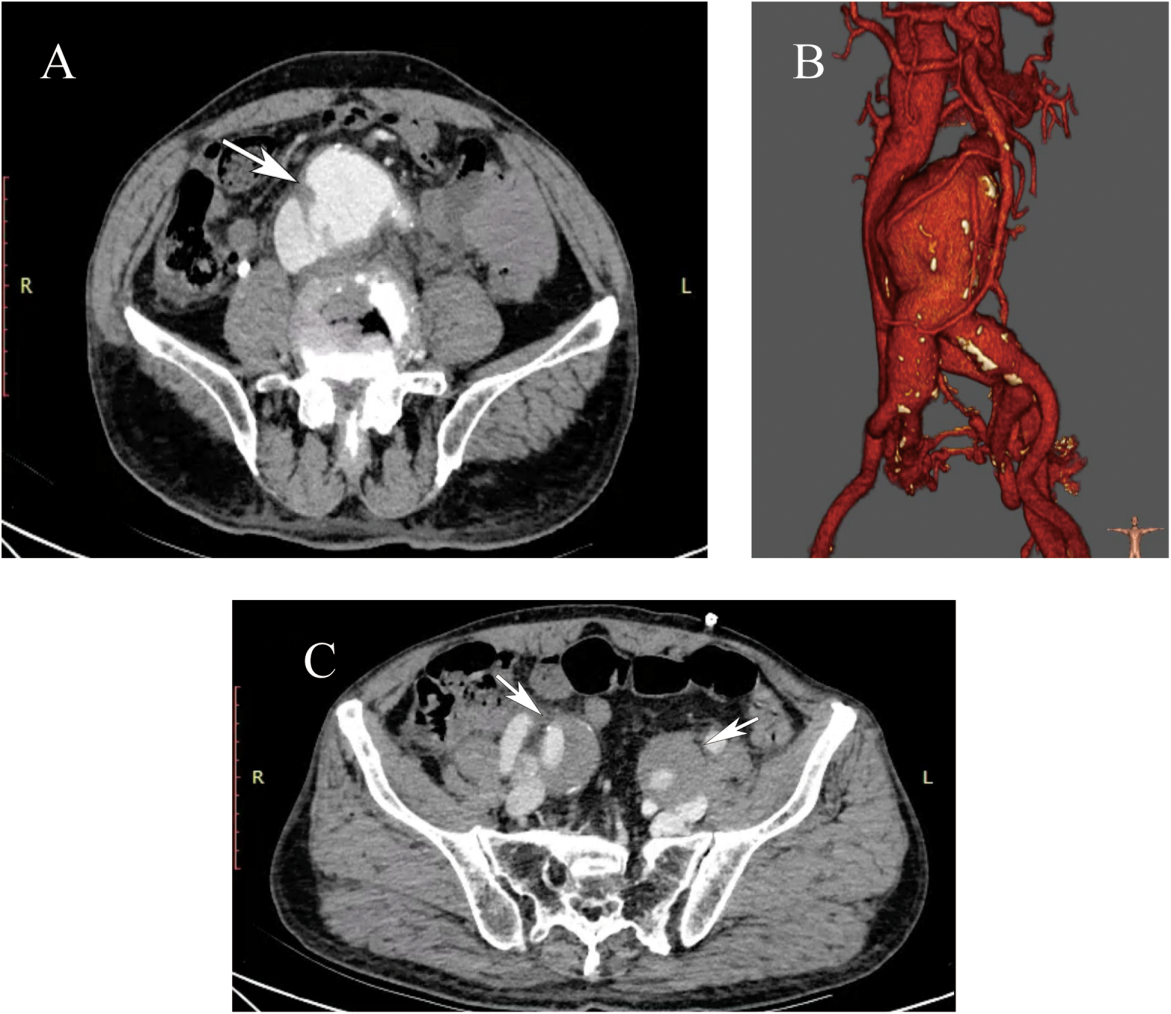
(A) The preoperative computed tomography angiography (CTA) showed a large abdominal aortic aneurysm (AAA) with associated aortocaval fistula (ACF, white arrowheads). (B) Three-dimensional reconstruction CTA showed an angulated aneurysm and tortuous aorto-iliac access. The maximum diameter of the AAA was approximately 8.7 cm. (C) The preoperative CTA showed bilateral internal iliac artery aneurysms (white arrowheads). The maximum diameter of the left internal iliac artery is approximately 32 mm, and the maximum diameter of the right internal iliac artery is approximately 34 mm.
